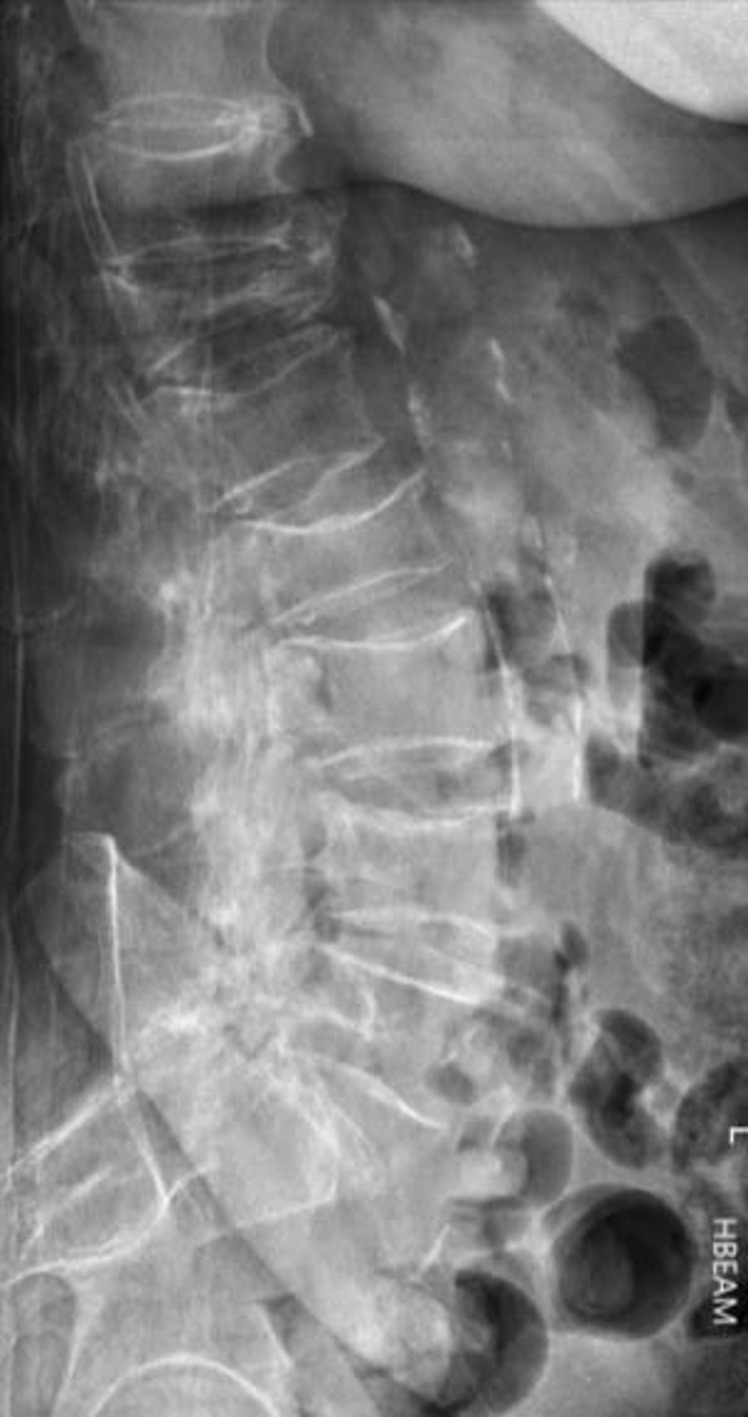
Lateral lumbar spine radiograph of an 80-year-old female with multiple insufficiency compression fractures; severe anterior wedge fracture at T12, mild compression fracture of L1 and L4 superior endplates and moderate compression fracture at L2.
